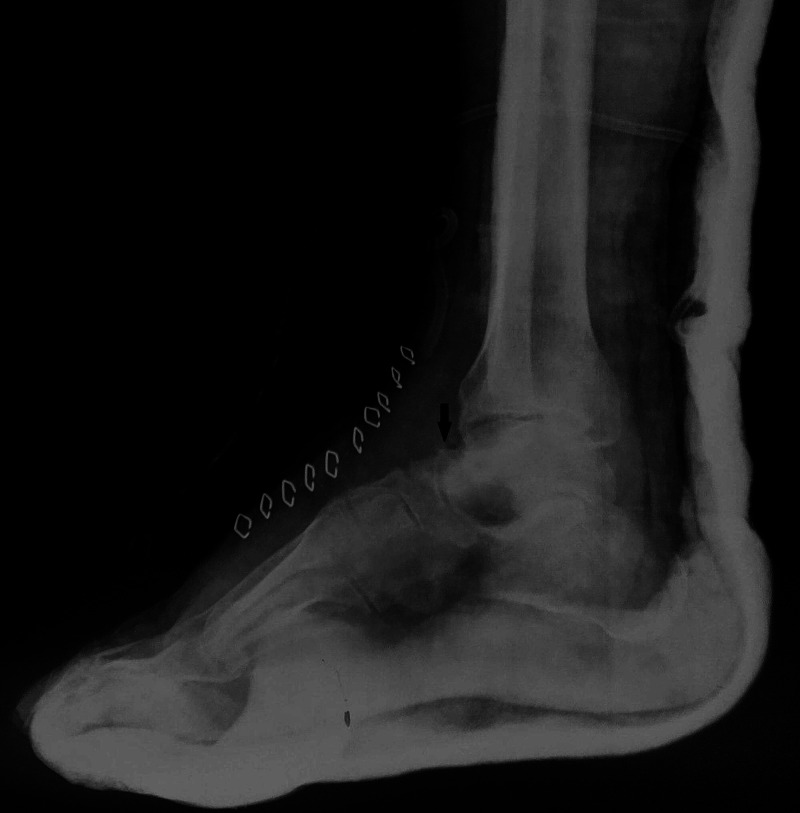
Post-operative lateral radiograph of the ankle and hindfoot demonstrates complete excision of the exophytic talus neck lesion (black arrow).
